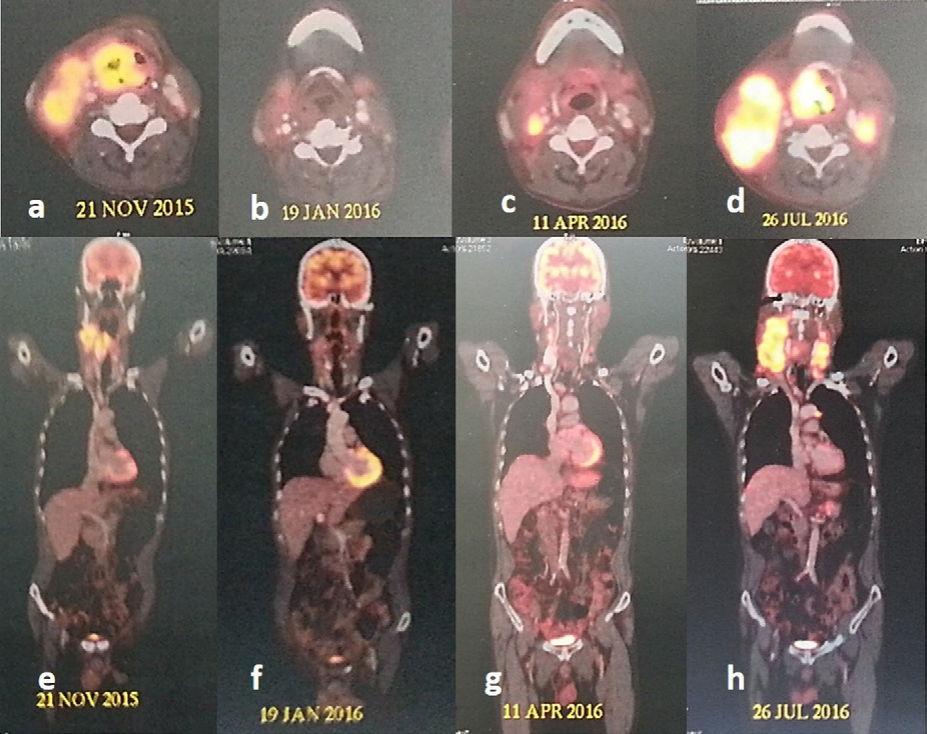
Serial whole-body PET-CT scans demonstrating the evolution of disease.
